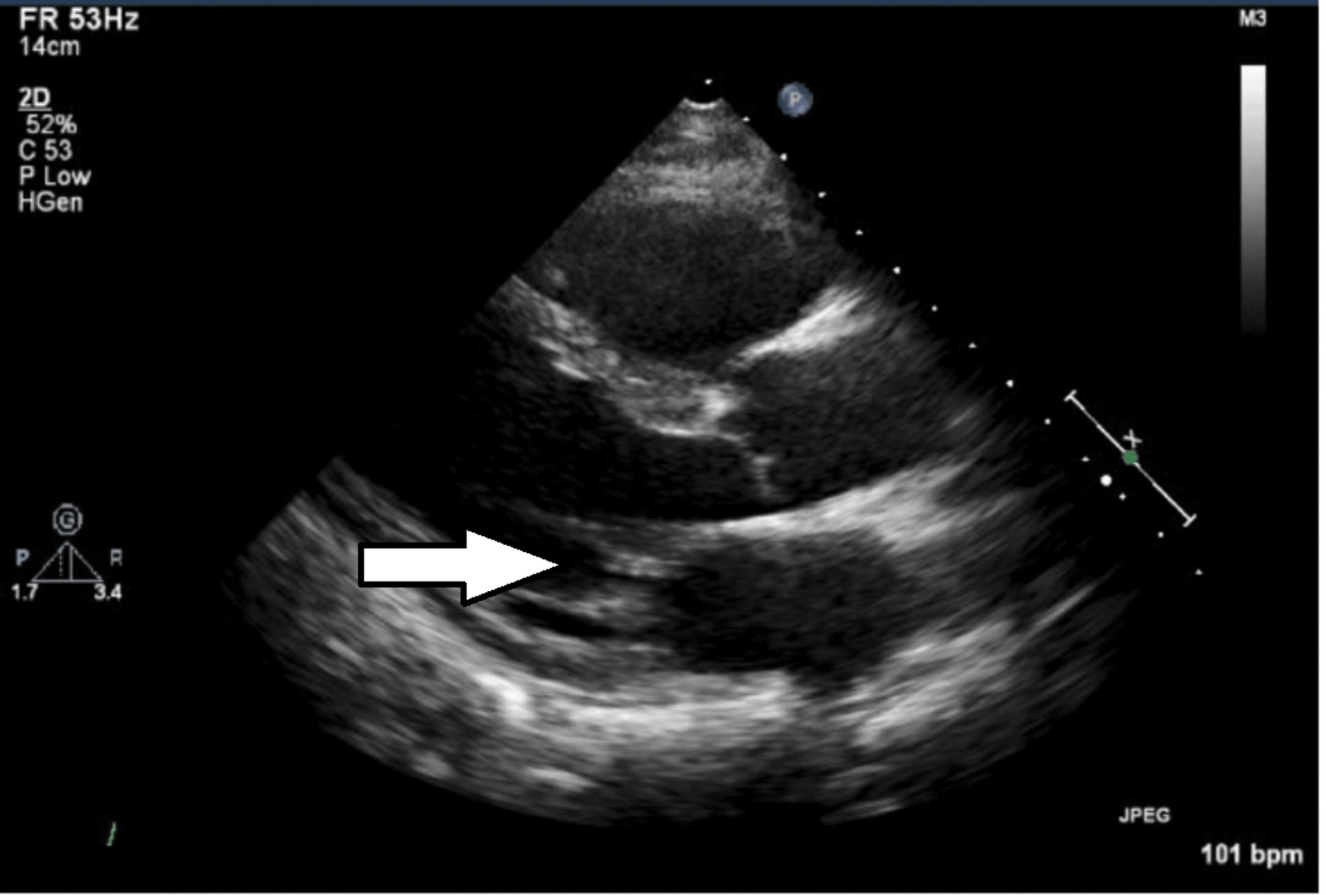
Transthoracic echocardiogram Parasternal long-axis view shows mild thickening of the mitral valve leaflets (arrows). No obvious vegetations or significant valvular regurgitation suggestive of infective endocarditis were observed.
